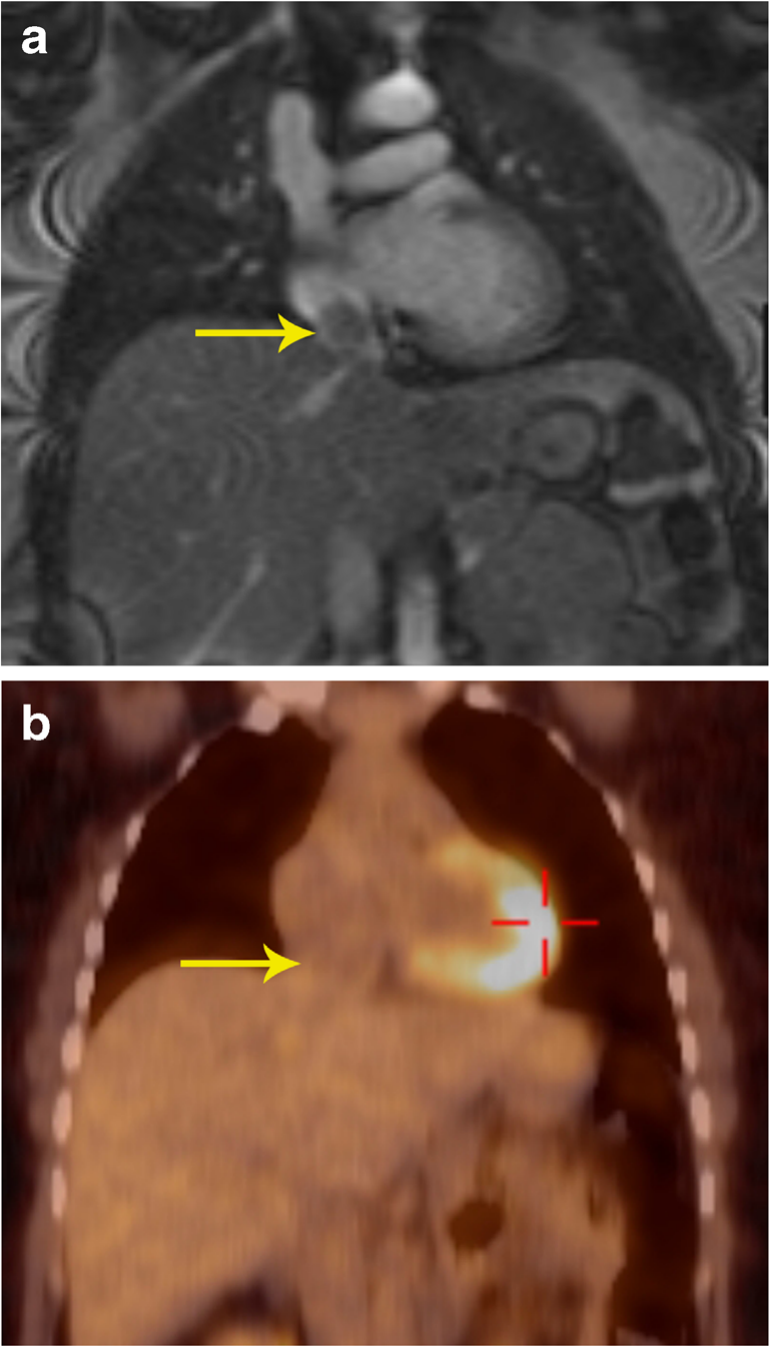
Characterization of mass. a Coronal first-pass perfusion MR image in a patient who presented with chest pain and IVC mass on echocardiography shows a mass that demonstrates contrast enhancement similar to the liver parenchyma. The mass also showed similar signal to liver in all other MRI sequences (not shown here). b Coronal FDG-PET/CT image shows no uptake in the lesion, indicating it is a benign process. This was proven to be a rare case of aberrant liver, which extended into the IVC
